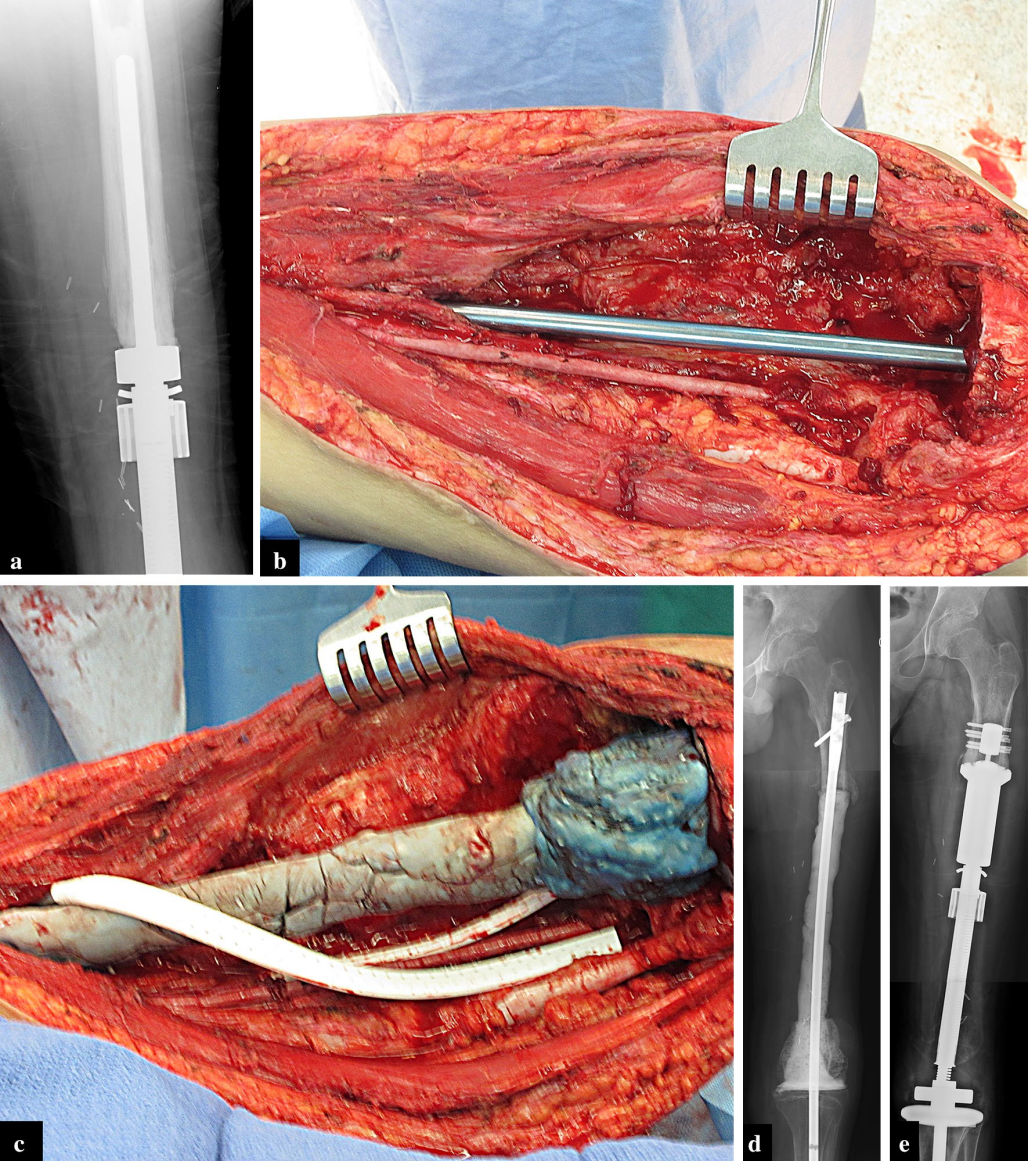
a Radiographic evidence of septic loosening of a femoral prosthesis. b, c Removal of the implant and placement of an antibiotic spacer consisting of an intramedullary nail (b) covered in antibiotic cement (c). d A radiograph of the antibiotic spacer after removal of the prosthesis. e Reimplantation of the prosthesis after eradication of the infection
